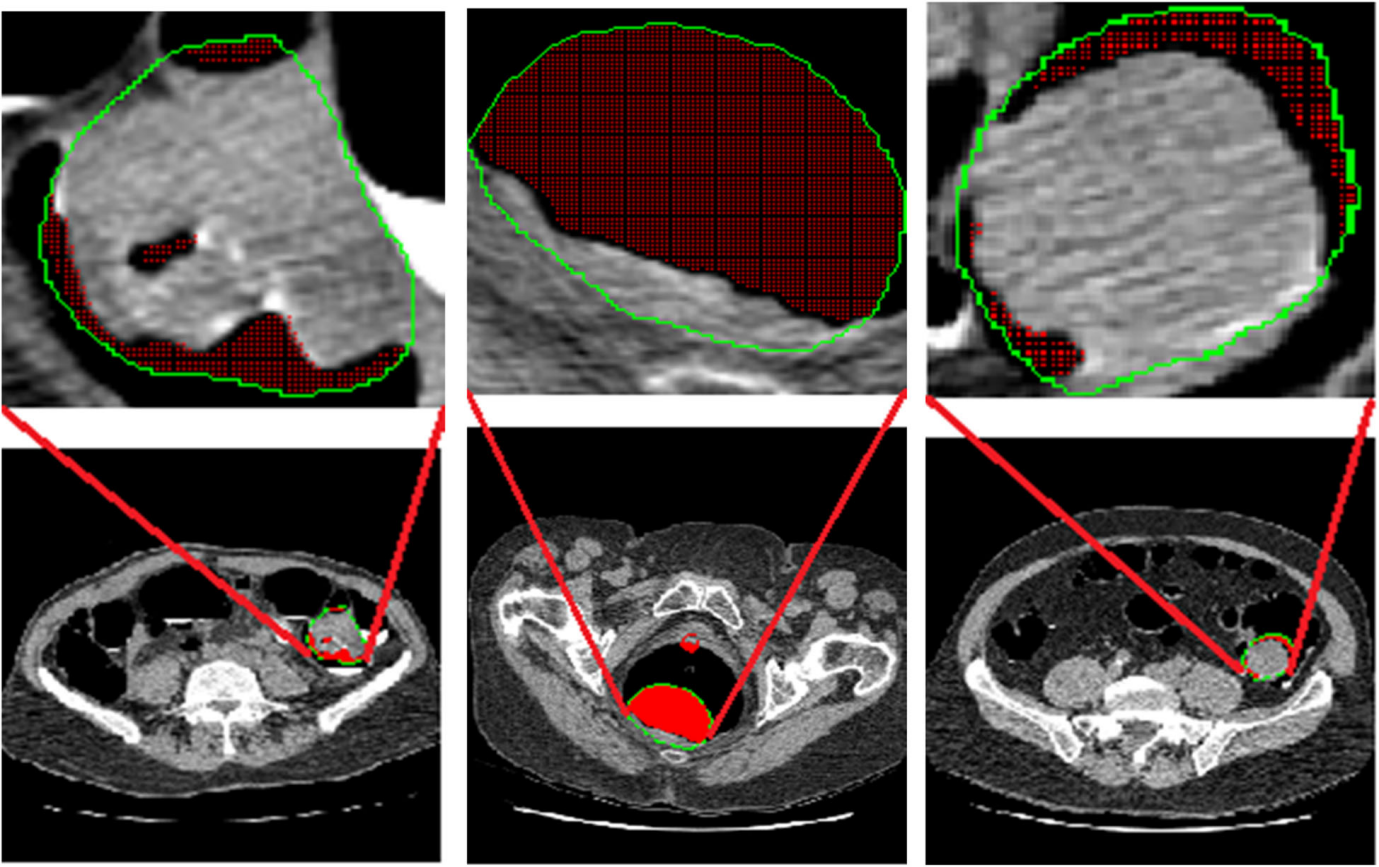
Polyp heterogeneity and texture in computed tomographic colonography. The green curves are their boundaries plotted by radiologists. The air in polyp is labeled by red color
